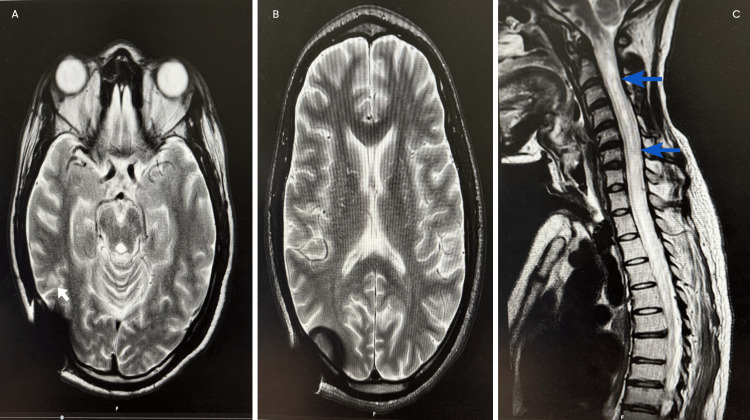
(A) Axial T2-weighted MRI demonstrating reduction in the size of a round hyperintense lesion in the left posterior cerebrum. (B) Axial T2-weighted MRI showing complete resolution of the left frontal white matter lesion. (C) Sagittal T2-weighted MRI of the spine showing a stable syrinx extending from C1 to C4. MRI: magnetic resonance imaging, white arrow: lesion in the left posterior cerebrum, blue arrow: syrinx
